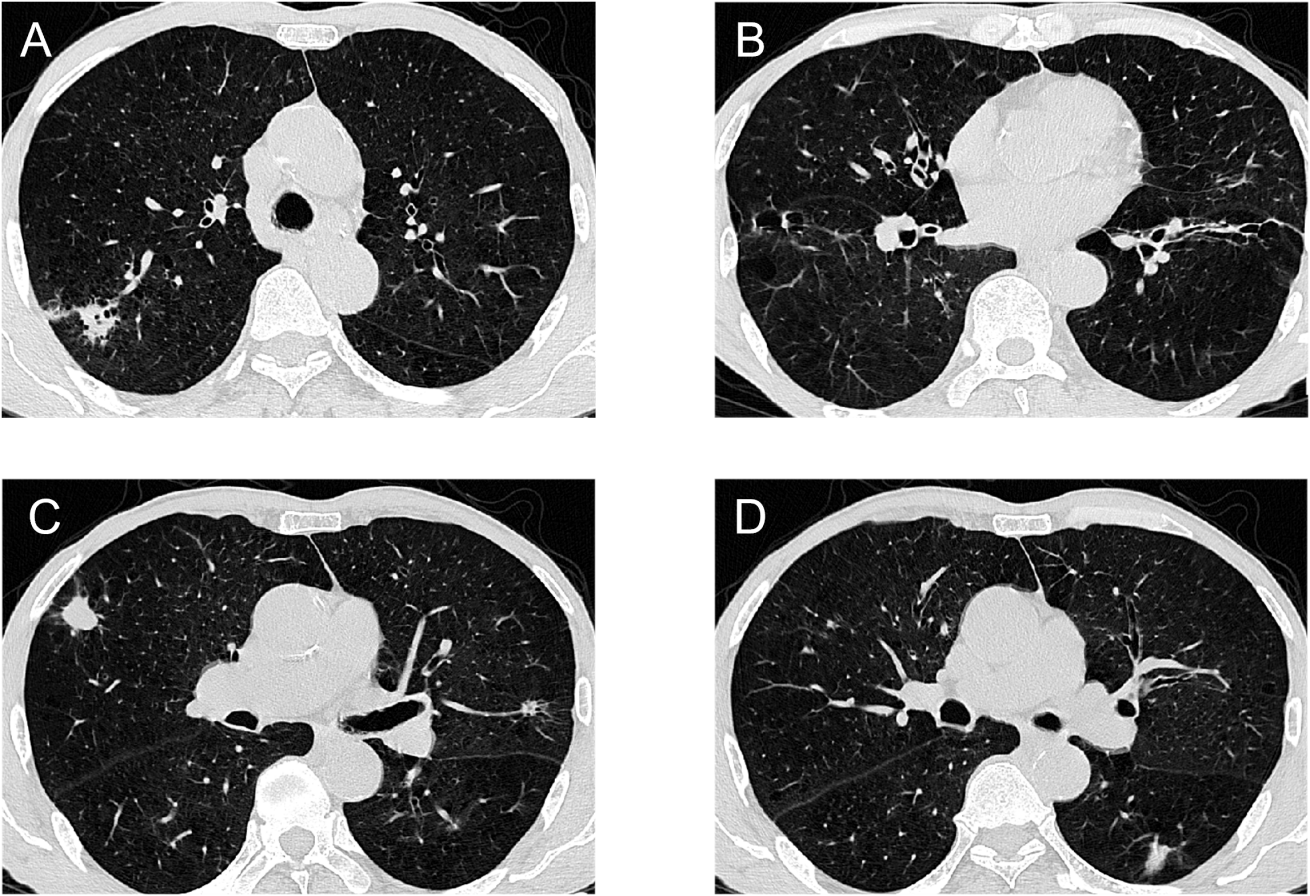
Chest CT results of the patient: (A)Right upper lung mass (B). Right lower lung mass (C)Left upper lung mass and right upper lung mass (D)Left lower lung mass.
